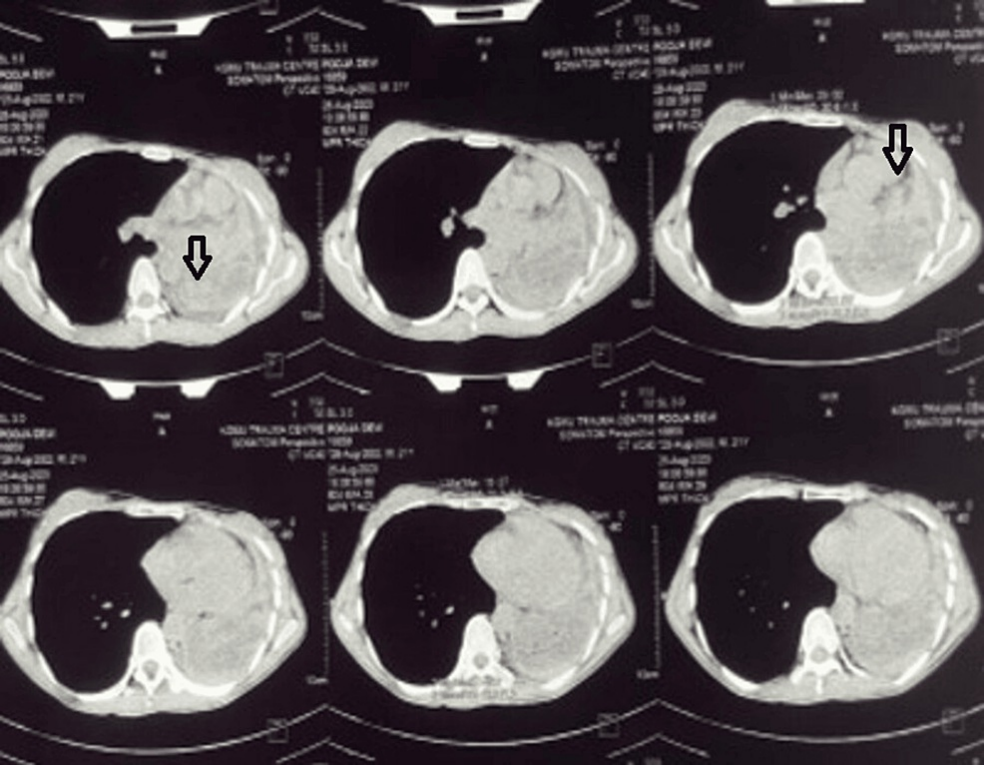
High-resolution computed tomography (HRCT) thorax showing left-sided space-occupying lesion infiltrating into the left main bronchus with collapse of the underlying lung parenchyma (arrow)
